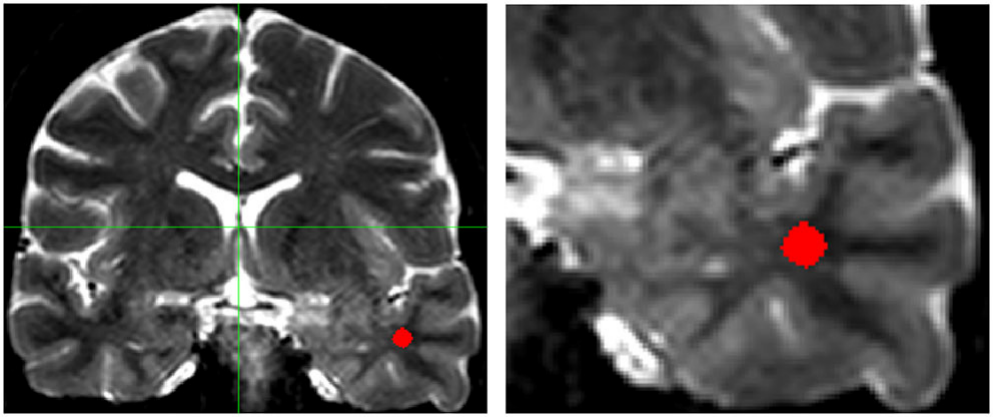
T2-weighted coronal multiecho images showing the localization of the region of interest (ROI) in the white matter of the temporal pole (Left: complete scan, Right: detail of the scan shown on the left). Areas of artifactual increase in T2-weighted hyperintense signal were carefully avoided. Images are in radiological convention.
